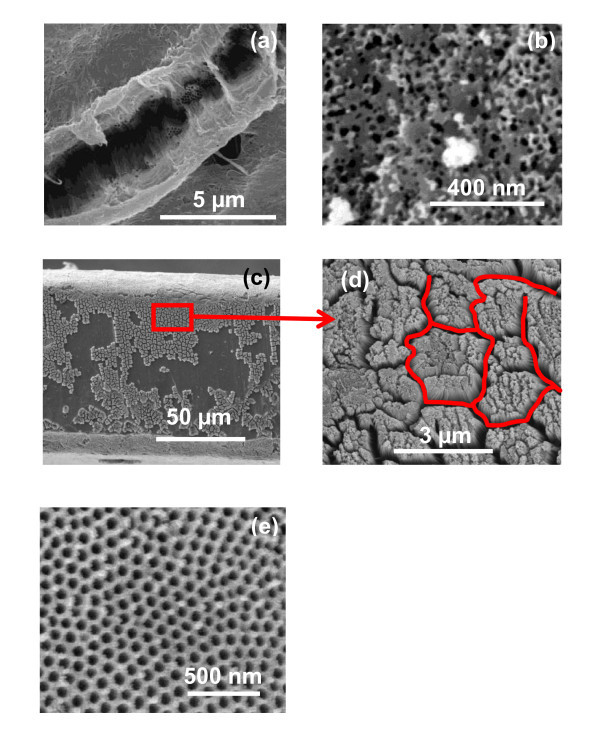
FESEM images of TNT fabricated in EG containing 0.5 wt% NH_4_F and 0.2 wt% H_2_O via single-step anodization for different times: (a) top surface view after 72 h of anodization, (b) top surface view after 11 h of anodization, (c) top surface view after ultrasonic agitation of 20 min in DI H_2_O, (d) magnified image of the marked area of (c), and (e) top surface view of TNP structure obtained after prolonged anodizing time (72 h) via second-step anodization in glycerol-based electrolyte.
